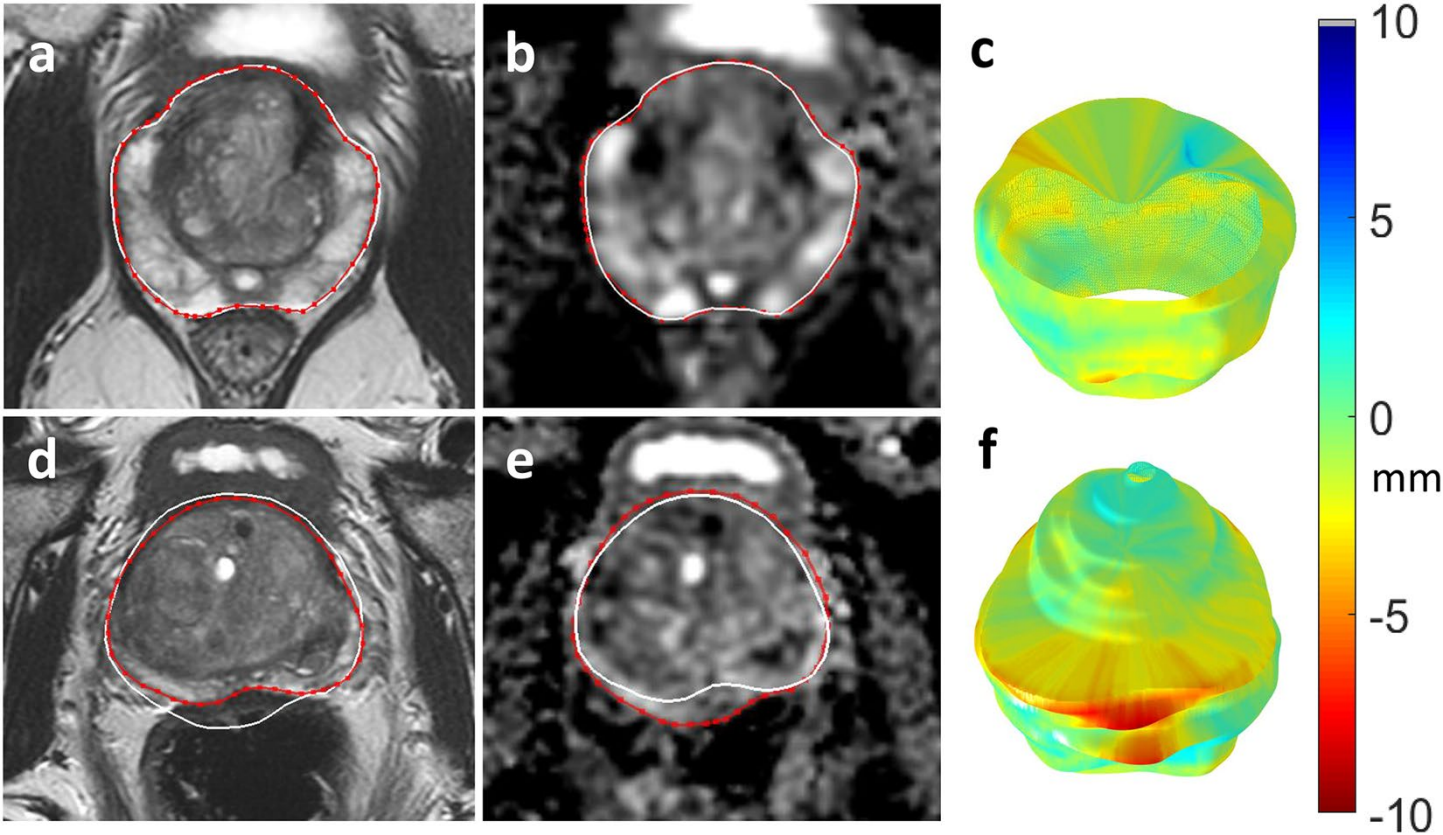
Distortion mismatch on anatomical images. 69 year-old patient on active surveillance with MRI showing no distortion on T2 (a) compared to diffusion-weighted imaging ADC maps (b); note collapsed rectum. (d,e) 68 year-old patient on active surveillance, ADC maps (e) show posterior distortion with warping compared to T2 imaging (d); note marked rectal loading. White outline of ADC map superimposed on red prostate outline on T2 image (and vice versa), to highlight the mismatch. Resulting surface distortion maps (c,f).
